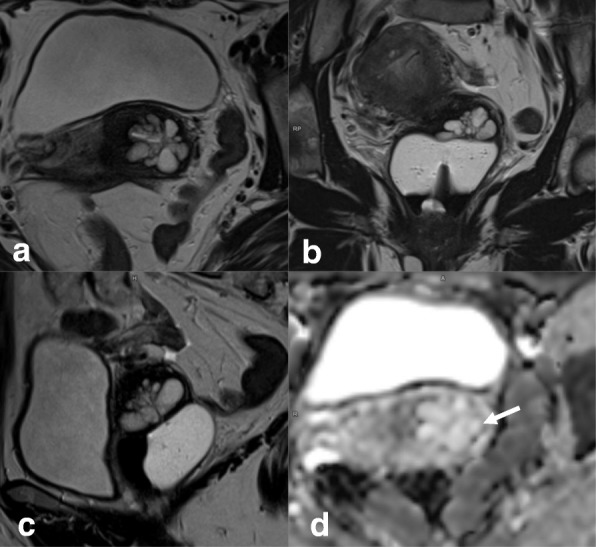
Tunnel clusters visualized by axial oblique T2WI (a), coronal T2WI (b), sagittal T2WI (c), and DWI-ADC map (d). Clustered cystic spaces have a round or oval appearance, an absence of invasion of the deep cervical stroma, and no diffusion restriction (arrow in d)
